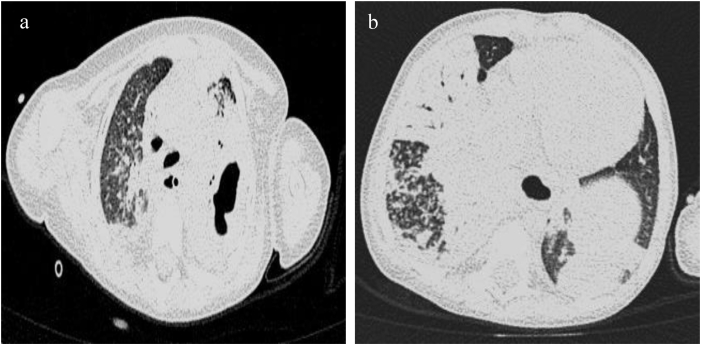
Atypical Covid-19 C T features. Unenhanced axial chest CT images showing segmental consolidations with no ground glass opacities, cavitation alongside with centrilobular and tree-in-bud nodules in an active tuberculous infection (a,b).
